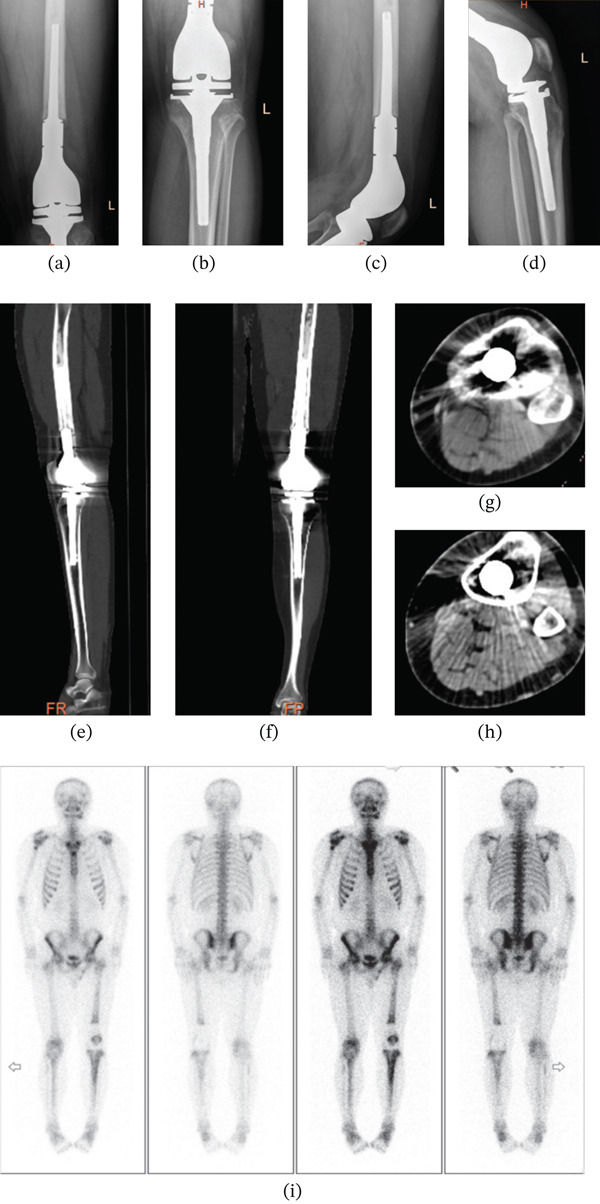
(a–h) DR and CT reexamination following surgery: Observations indicate changes after the absence of the lower left femur and the implantation of an artificial knee joint, which are largely consistent with preoperative findings; (i) postoperative whole‐body bone static imaging reveals a focal abnormal metabolic increase in the upper segment of the original right humerus, similar to previous assessments, suggesting a benign lesion. The reduced metabolic activity at the lower end of the left femur, along with increased abnormal metabolism in both the knee joint and middle to upper segments of the left tibia, exhibited greater stability compared with prior evaluations. These changes posttumor resection and prosthesis implantation were considered in conjunction with the patient′s medical history.
